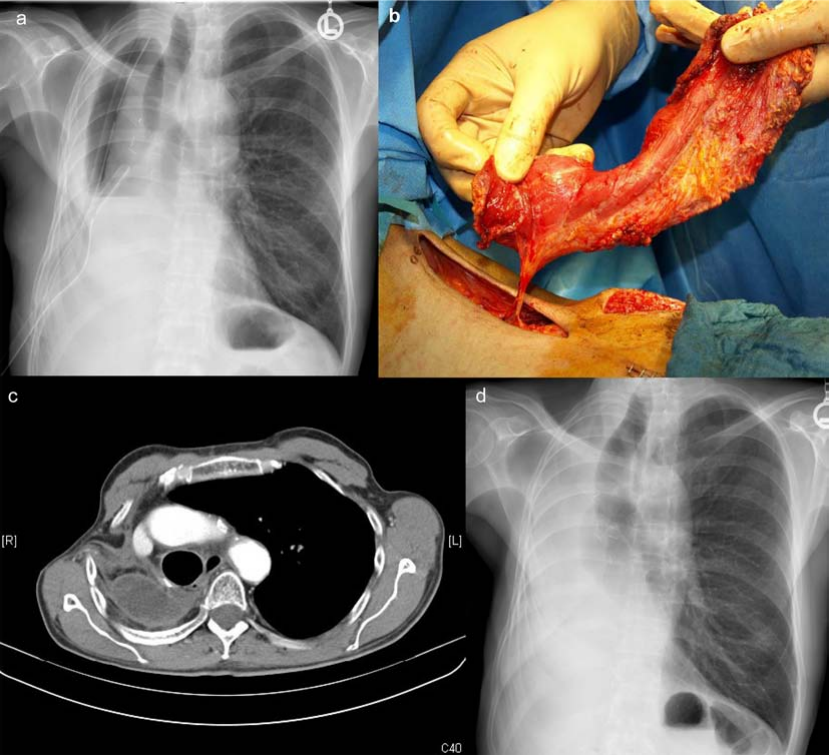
Images demonstrating the progress of treatment: (a) X-ray of the chest demonstrating thickened right-sided thoracic wall and two drains, the day before open debridement; (b) preparation of the pedicled latissimus dorsi muscle flap; (c) CT-scan 3 months after definitive closure demonstrating adequate shift of the mediastinum to the right side and the entrance of the muscle; (d) corresponding chest X-ray after 3 months.
